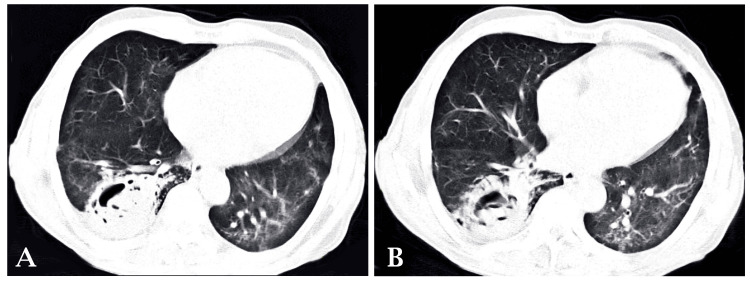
Computed Tomography Scan of the Chest of the Patient at Presentation (A) evidence of crescent-shaped lucency around the soft tissue and (B) Posterobasal segment of the right lower lobe with adjacent areas of ground glass opacities and soft tissue opacification within the cavity.
